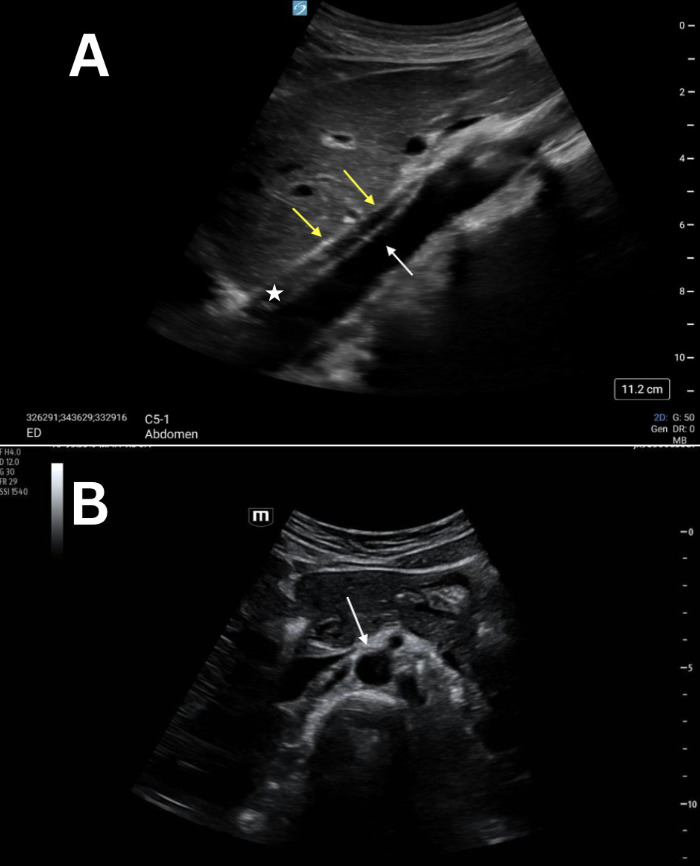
Grayscale point of care ultrasound (POCUS) images of a 27-year-old female standardized patient showing (A) sagittal view with two neighboring linear, hyperechoic structures (double yellow arrow and white arrow) and the intervening hypoechoic crus of the diaphragm (white star) and (B) transverse view with a normal, anechoic lumen of the abdominal aorta (white arrow).
